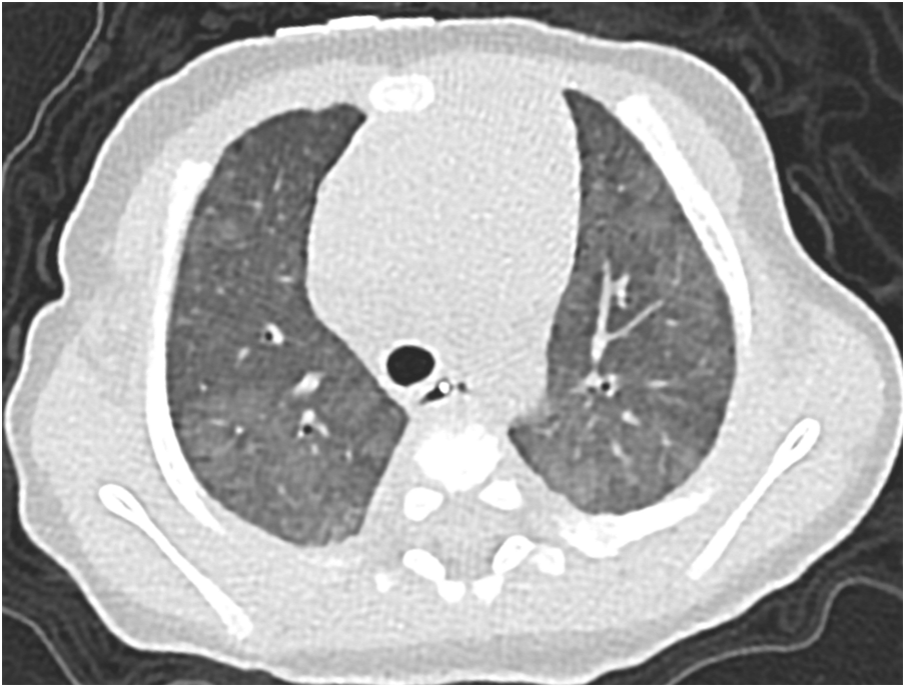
Case 1: axial CT images of lung window settings at 3 months. Axial CT images show heterogeneous ground-glass opacities in both lung fields.
